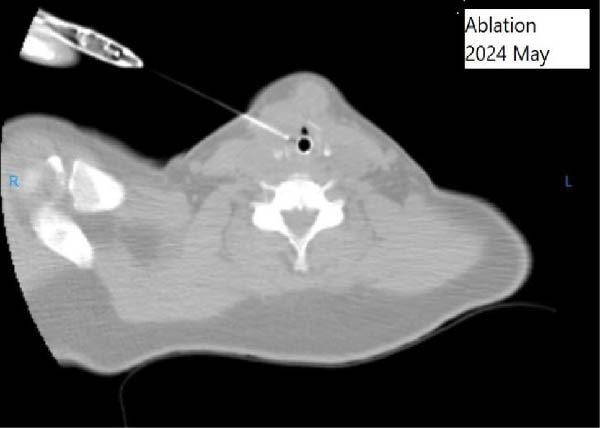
During RFA, CT‐guided ablation of the laryngeal mass.
